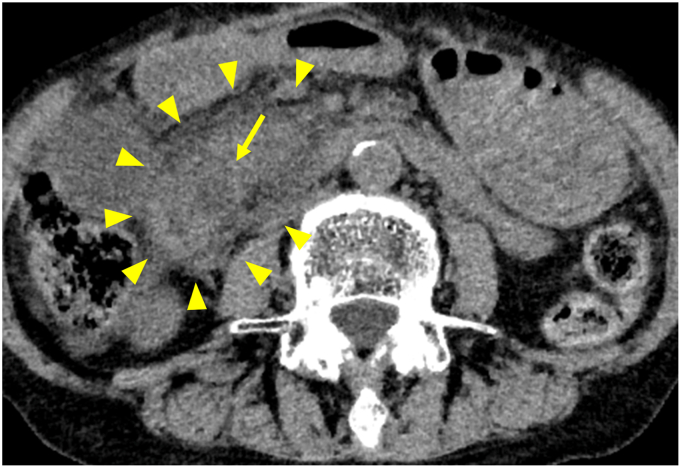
CT image showing swelling of the pancreatic head (*arrowheads*) and dilation of the common bile duct (*arrow*). These findings are suggestive of cholangitis and pancreatitis secondary to postendoscopic papillectomy stricture.
